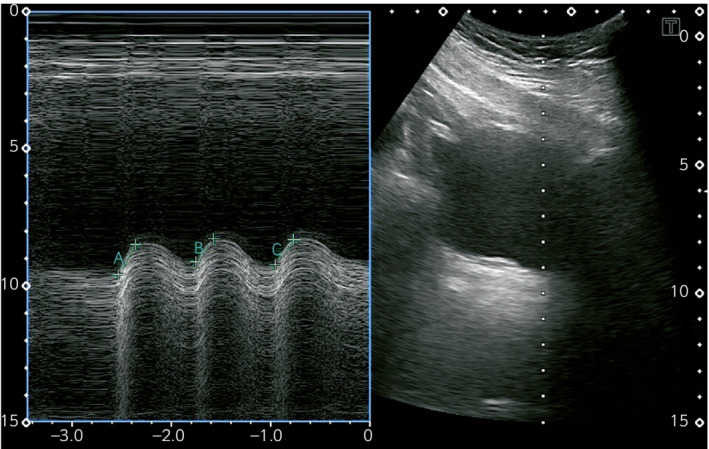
M‐mode ultrasound imaging. The bladder is displayed in the median sagittal imaging plane. The M‐mode cursor is set to the bladder base, which moves most at PFM contraction. Bladder base elevation time, length, and speed during PFM contraction are measured by using M‐mode ultrasound system. Bladder base elevation is measured at the highest point from the start point of PFM contraction.
